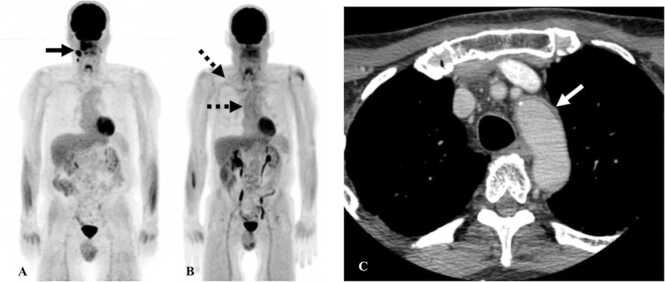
Immune-mediated vasculitis in a 67-year-old man with squamous cell cancer of the oropharynx on Nivolumab. A. Reconstructed MIP image from PET/CT shows FDG-avid lesion in the floor of mouth with lymphadenopathy (black arrow). B. Reconstructed MIP image from PET/CT obtained 6 months after initiating Nivolumab therapy shows resolution of the lesion with new FDG uptake along the walls of the aorta and subclavian arteries consistent with vasculitis (black dashed arrow). C. Contrast-enhanced CT of the neck from the same day shows wall thickening of the arch of aorta (white arrow).
